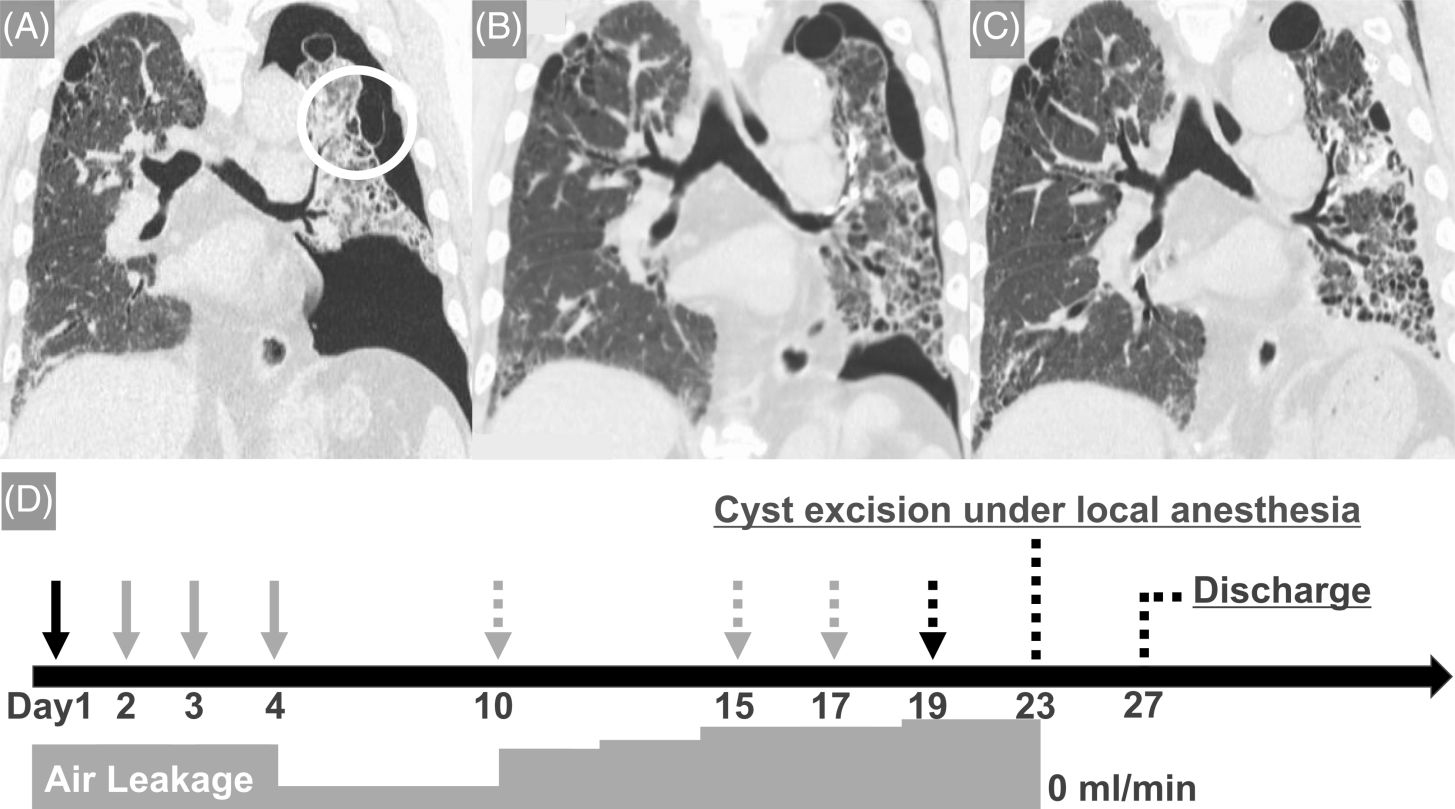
(A) On the day of admission, CT revealed the left pneumothorax. The white circle indicates a cyst with a suspected leak. (B) On day 17, CT revealed lungs without expansion after the replacement of Endobronchial Watanabe Spigot (EWS). (C) On day 23, CT revealed an expanded lung obtained after the operation. (D) A summary of the patient's clinical course. The black arrow: thoracic drain placement. The grey arrow: pleural blood patching. The grey dotted arrow: replacement of EWS into apicoposterior segment bronchus (B1/2). The black dotted arrow: left thoracography. CT, computed tomography.
